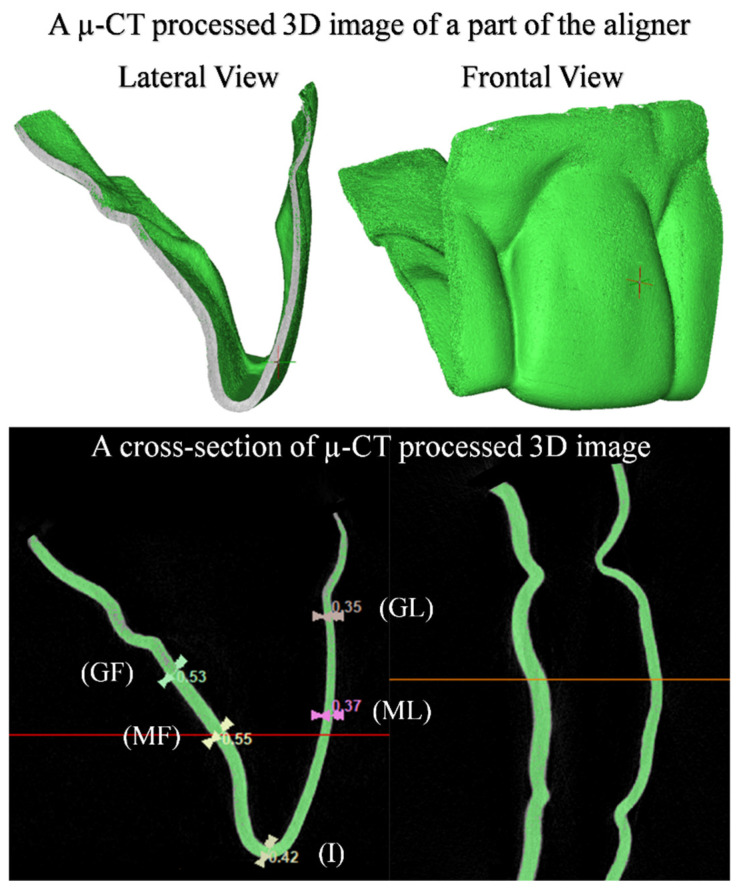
A µ-CT processed 3D image for a part of the aligner over the upper right central incisor (Tooth 11) for measurement of aligner thickness by MIMICS 25.0 software, at various reference points (gingivo-facial (GF), mid-facial (MF), incisal (I), mid-lingual (ML), gingivo-lingual (GL)).
